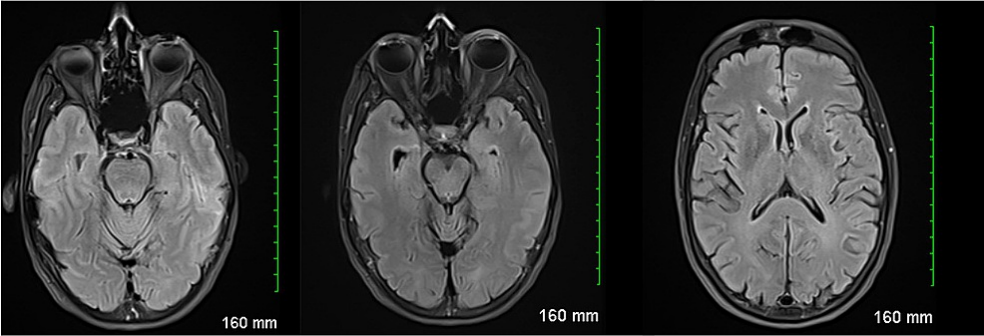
MRI of the brain is normal
